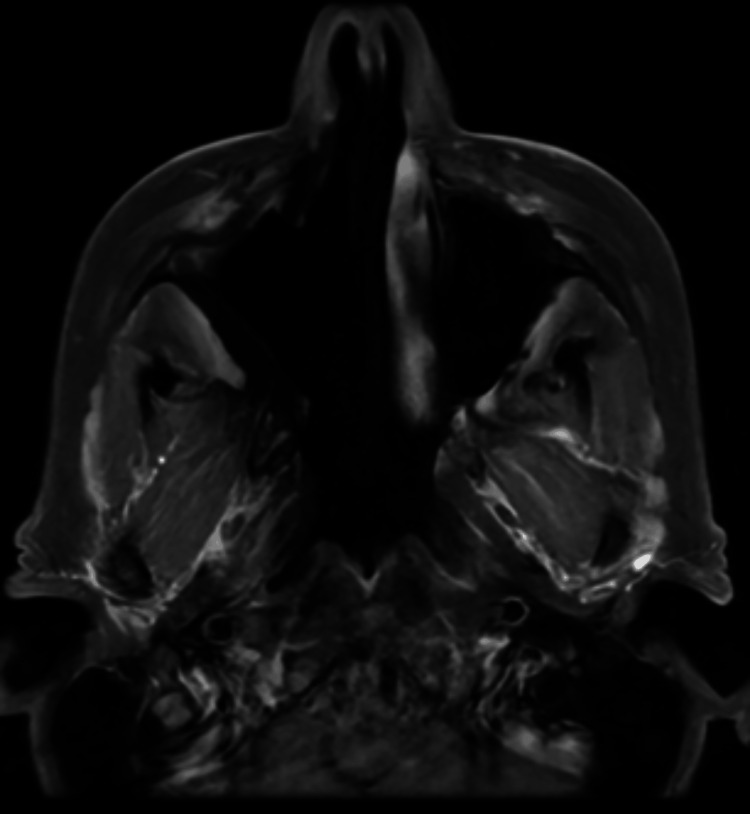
Post-gadolinium T1 weighted MRI in August 2023 (axial view) Post-treatment inflammation without any residual tumour.
